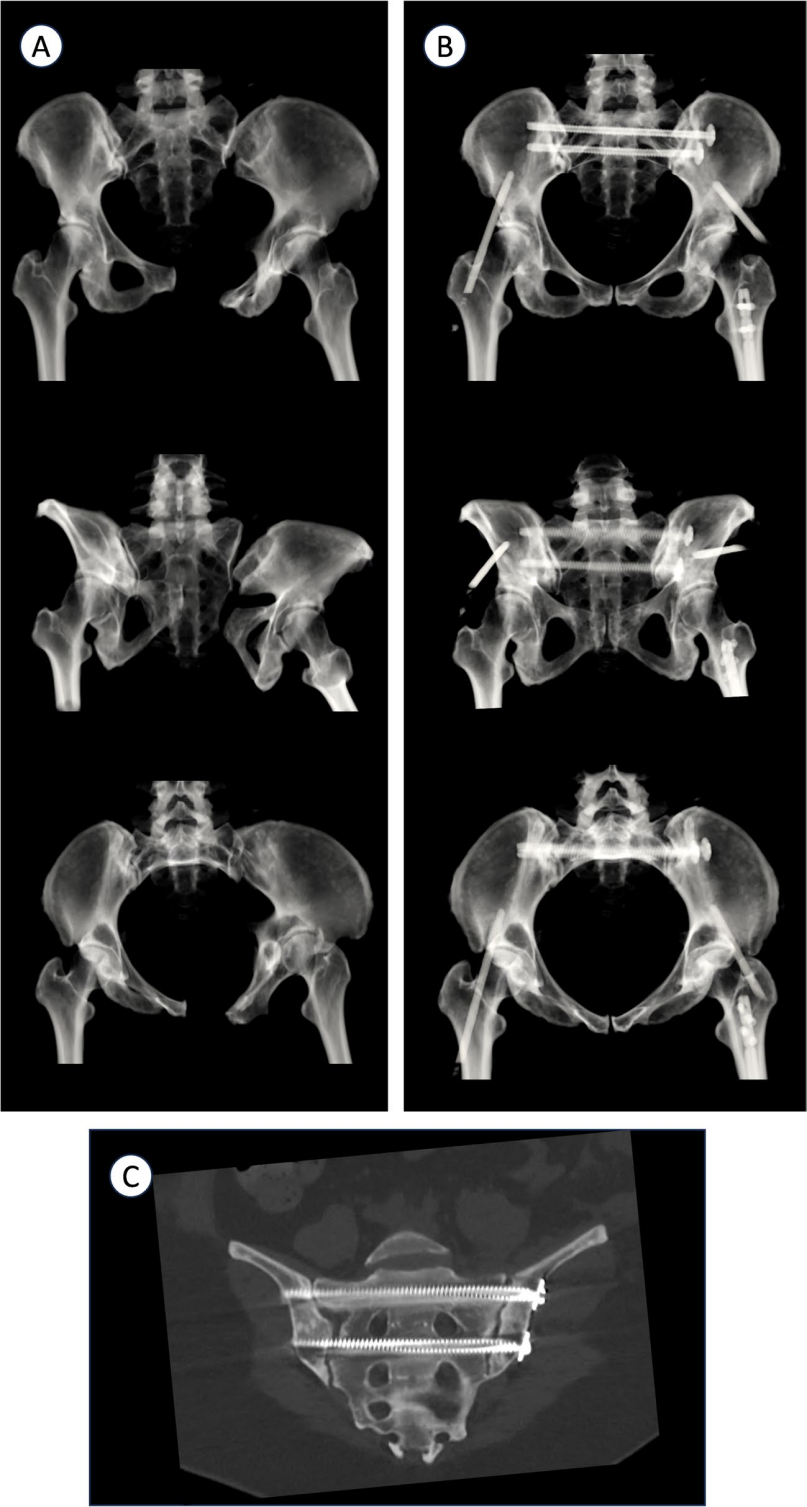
Postoperative CT scan demonstrating reduction and screws trajectories
